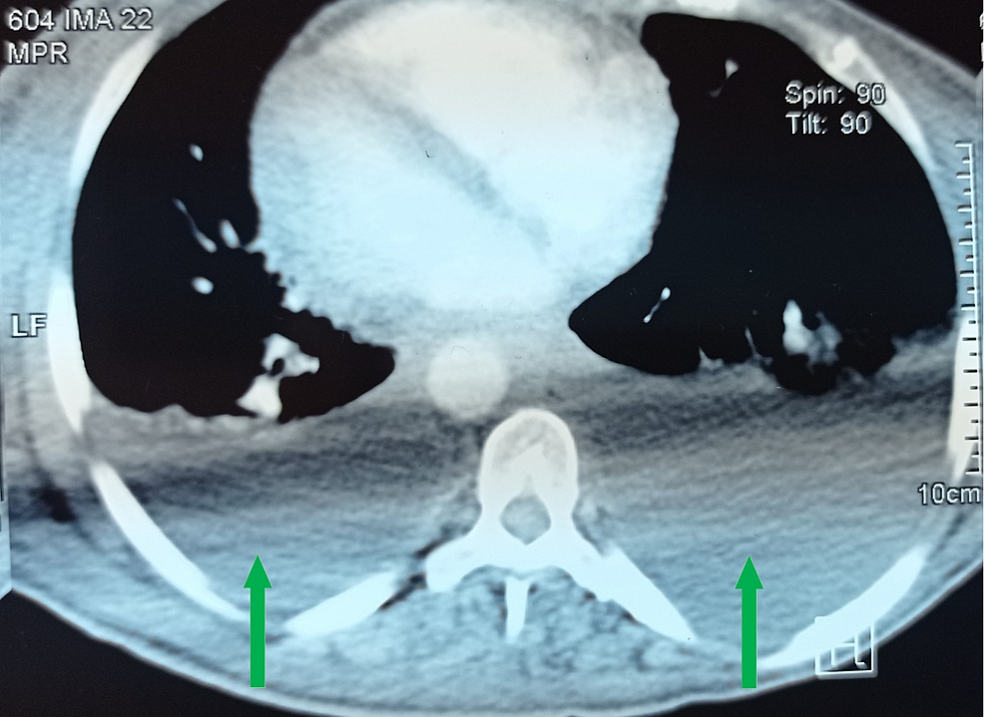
Cross-sectional thoracic CT scan showing bilateral pleural effusion (green arrows). CT: Computed Tomography
